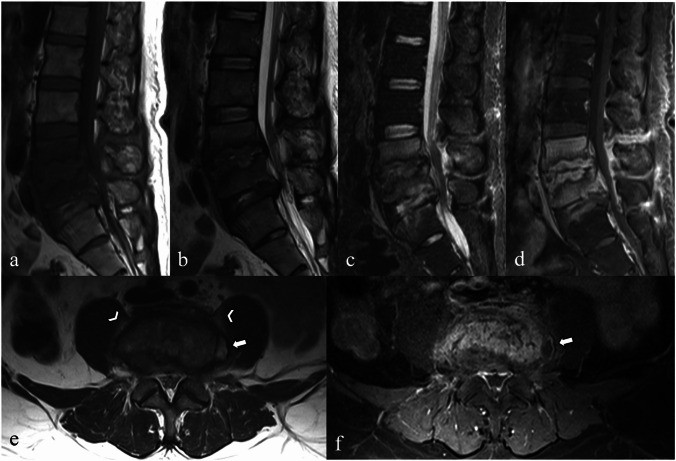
Magnetic resonance images of a 37-year-old male with pyogenic spondylitis caused by *Streptococcus agalactiae*. Sagittal T1-weighted (a), T2-weighted (b), short tau inversion recovery (STIR) (c), and fat-suppressed contrast-enhanced T1-weighted (d) images show hypointense T1-weighted and hyperintense T2-weighted/STIR signals with enhancement of the severely destroyed L4-L5 intervertebral disc, partially destroyed L5-S1 disc, and the surrounding L4, L5, S1 endplates. Edematous, enhancing marrow involving the entire L4 and L5 vertebral bodies is also noted. Axial T2-weighted imaging at the L4–L5 level (e) demonstrates the imaging psoas sign, characterized by T2 hyperintensity within the bilateral psoas muscles (arrowheads). Axial fat-suppressed contrast-enhanced T1-weighted image (f) shows enhancement of the perivertebral inflammatory soft tissue and a small abscess in the left paravertebral region (arrows)
